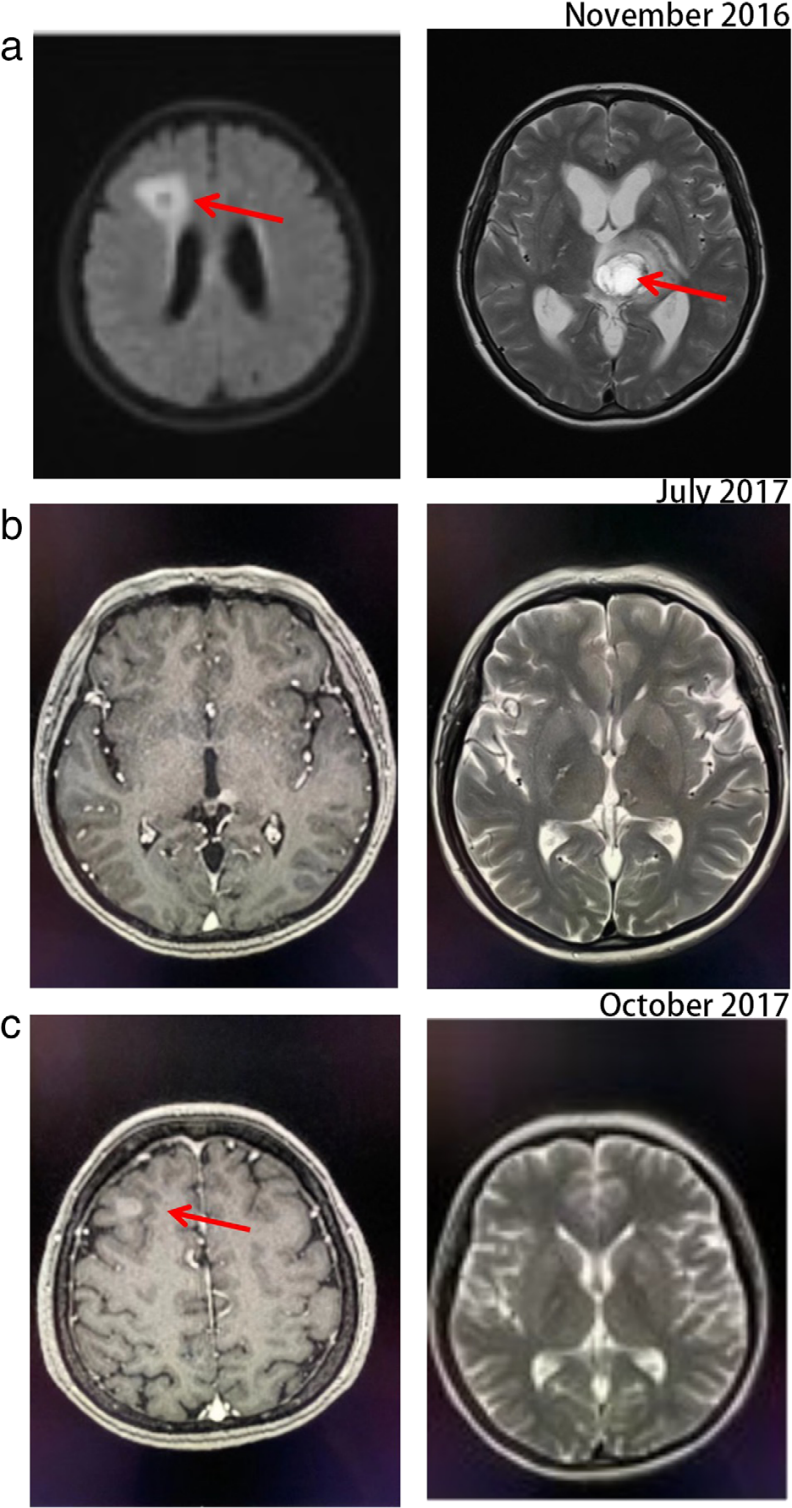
Magnetic resonance imaging (MRI) of the patient's metastatic brain tumor lesion (a) before brigatinib treatment (November 2016), (b) response to brigatinib (July 2017), and (c) resistant to brigatinib (October 2017). The red arrows indicate the brain lesion
